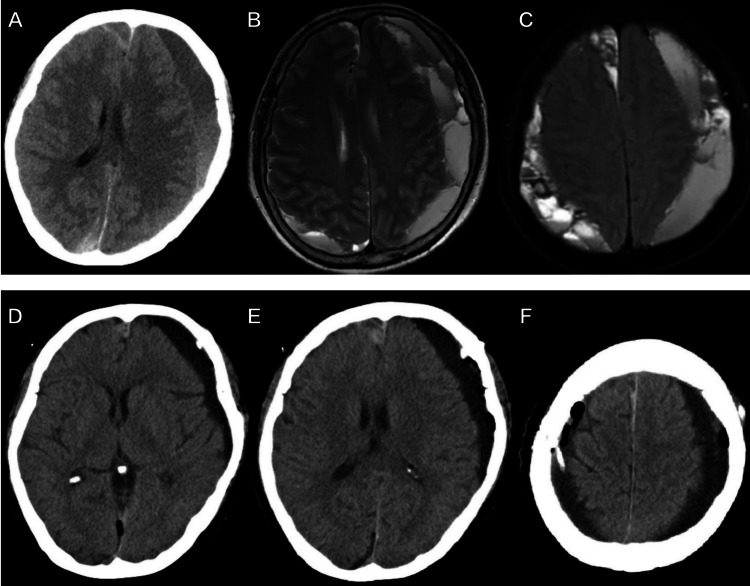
Preoperative CT and MRI axial brain images. Upper row (A, B, C): Preoperative neuroimaging showing bilateral chronic subdural hematoma, wherein the right hematoma is confined to the parietal lobe and the left hematoma is a typical hematoma covering the frontoparietal area, with a midline shift of 8.7 mm. Lower row (D, E, F): Postoperative CT (day two) demonstrating a left low-density shadow with a midline shift of 4.3 mm.
